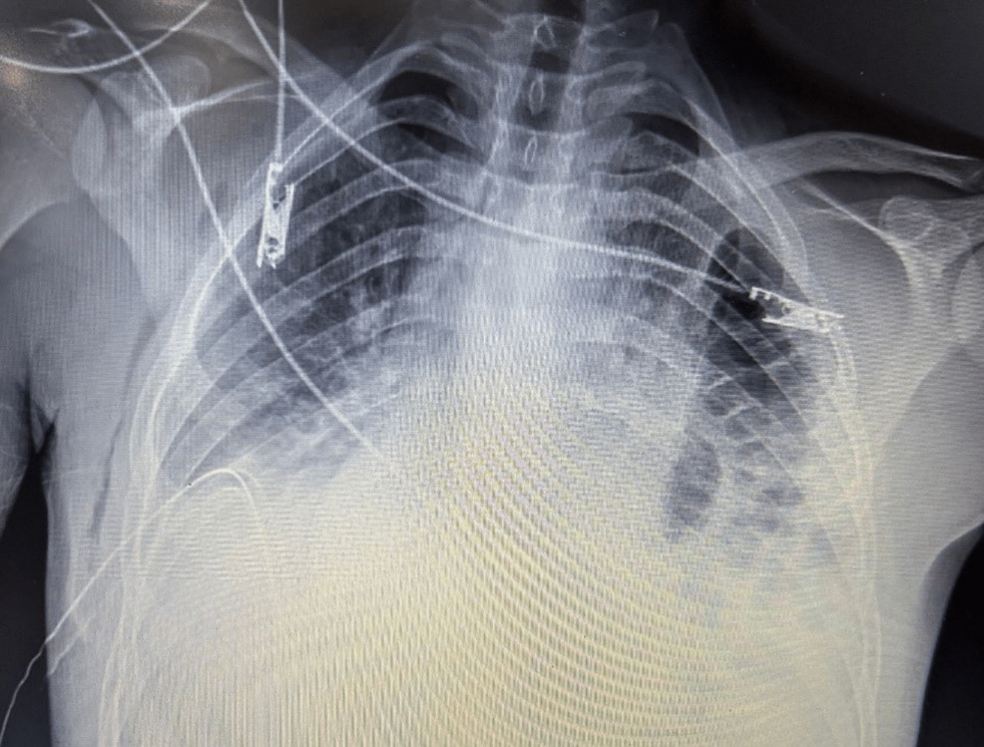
Chest X-ray showing collapsed left lung with bowel loops seen in the left hemithorax.
